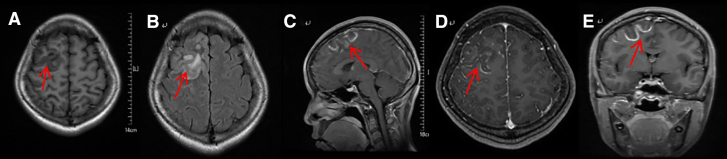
(A) The T1WI sequence of the transverse cranial section; (B) The T2WI sequence of the transverse craniocerebral section; (C) T1WI sequence of the cranial magnetic resonance imaging enhances the sagittal position; (D) Enhanced transverse position of T1WI sequence in cranial magnetic resonance imaging; (E) T1WI sequence of the cranial MRI enhances the coronal position. MRI = magnetic resonance imaging, T1WI = T1-weighted imaging, T2WI = T2-weighted imaging.
